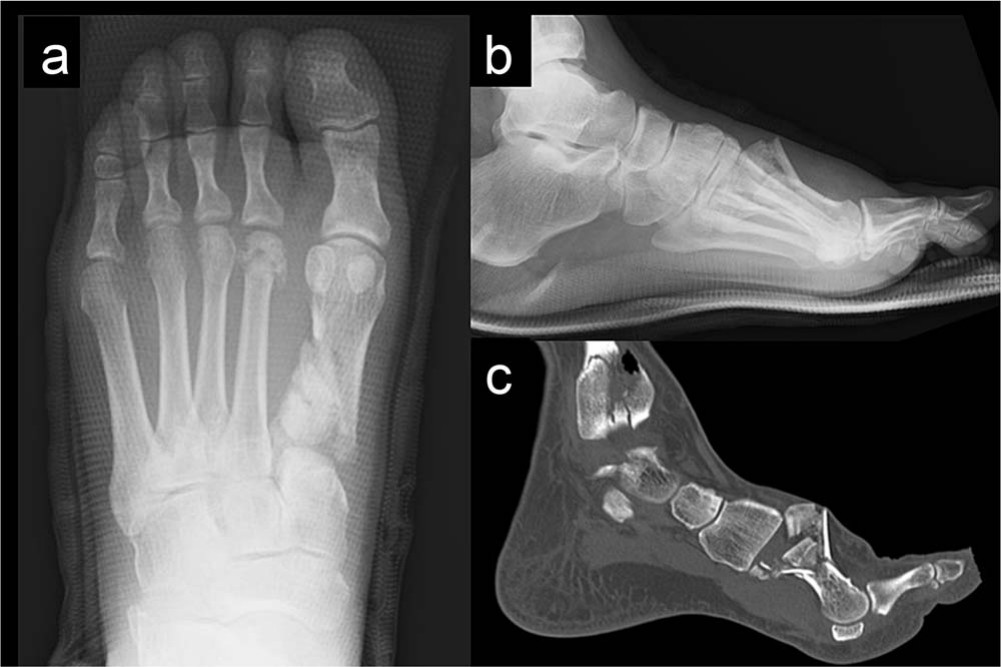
Radiography (a, b) and computed tomography (c) images of the left forefoot showing the comminuted first-metatarsal fracture.
